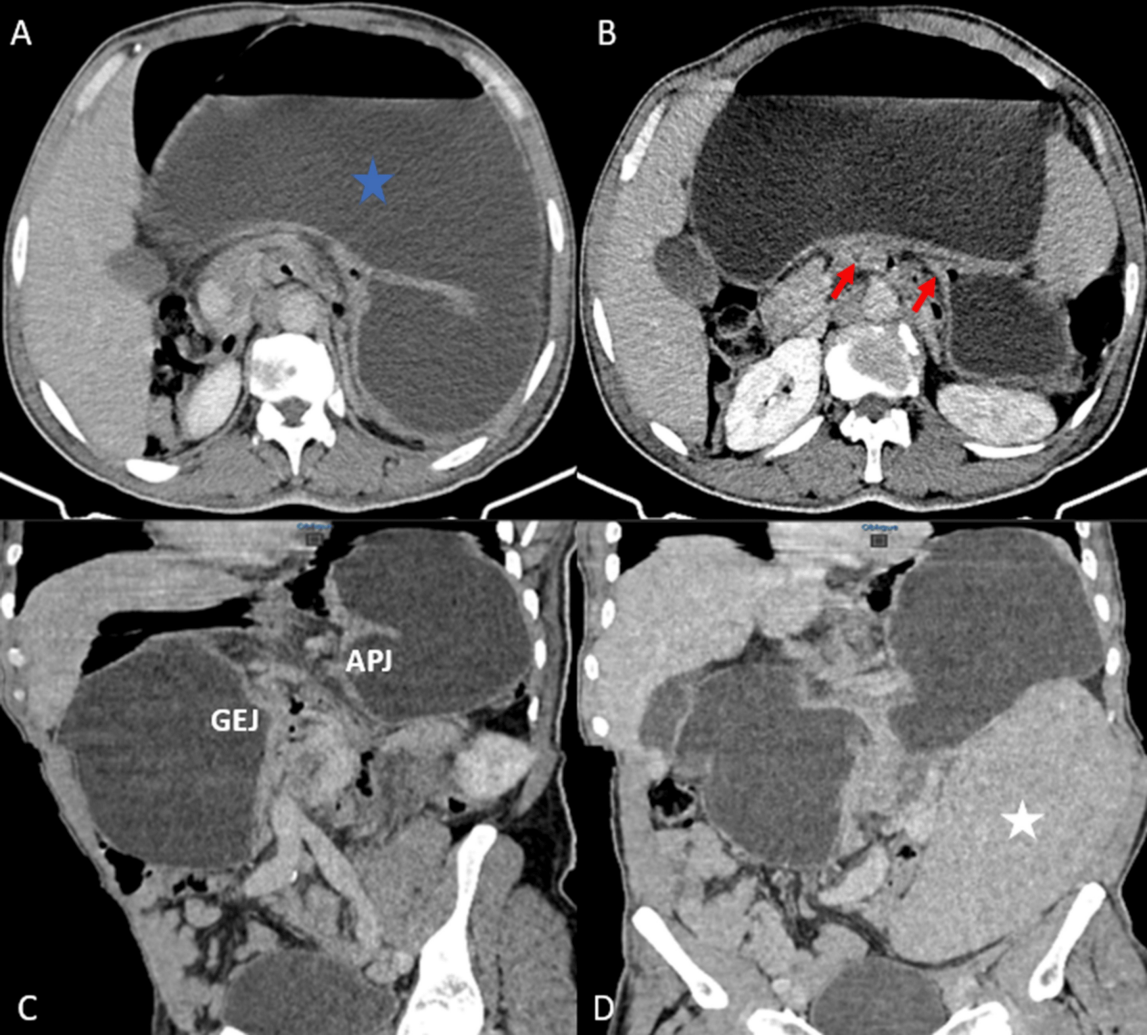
Contrast-enhanced abdominal CT findings. Grossly enlarged stomach (A, blue star). The stomach is twisted mesenteroaxially, showing the typical double bird beak sign (B, red arrows), an upside-down-looking stomach (C) with the APJ positioned higher than the GEJ. The enlarged spleen is positioned in the left lumbar and iliac region (D, white star). APJ: antropyloric junction, GEJ: gastroesophageal junction
